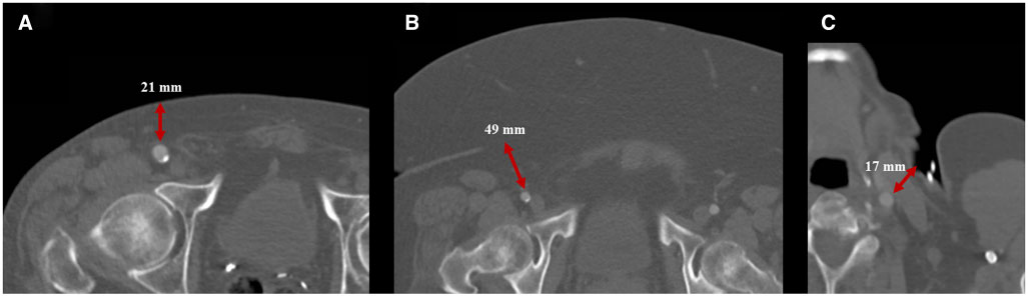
Features associated with transfemoral (A and B) and transcarotid (C) access. (A) Computed tomography image displaying an axial plane at the level of the right femoral artery in a normo-weight patient with a body mass index of 25.3 kg/m^2^. The distance between the skin and the anterior wall of the femoral artery was 21 mm (double-head red arrow) and no abdominal adipose panicle was observed. (B) Computed tomography image displaying an axial plane at the level of the right femoral artery in a morbid obese patient with a body mass index of 42.5 kg/m^2^. The distance between the skin and the anterior wall of the femoral artery was substantial (49 mm, double-head red arrow), and a huge abdominal adipose panicle was observed. (C) Computed tomography axial plane of the left common carotid artery, demonstrating its superficial location. BMI: body mass index; TC: transcarotid; TF: transfemoral.
